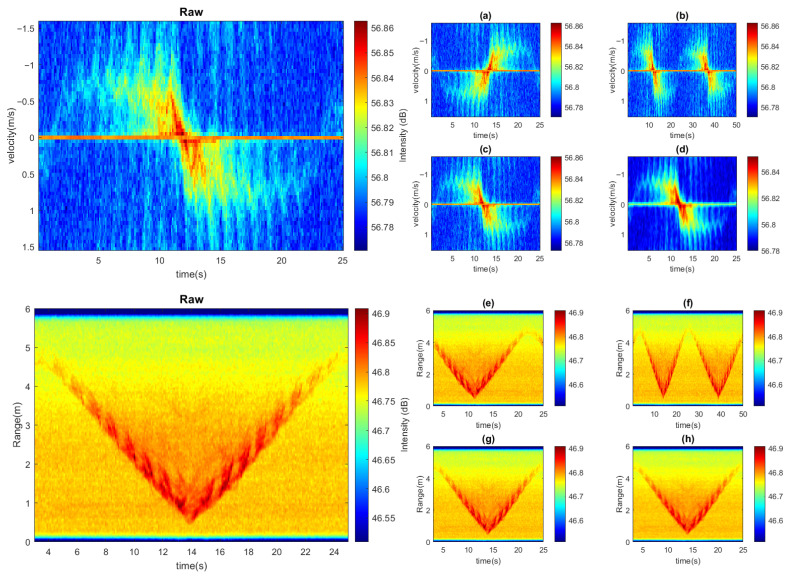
The data augmentation and imaging blurring: Range data: (a) mirror image, (b) twice the length of the time window, (c) Gaussian blur σ=0.4, (d) Gaussian blur σ=0.8; Doppler data: (e) mirror image, (f) twice the length of the time window, (g) Gaussian blur σ=0.4, (h) Gaussian blur σ=0.8.
